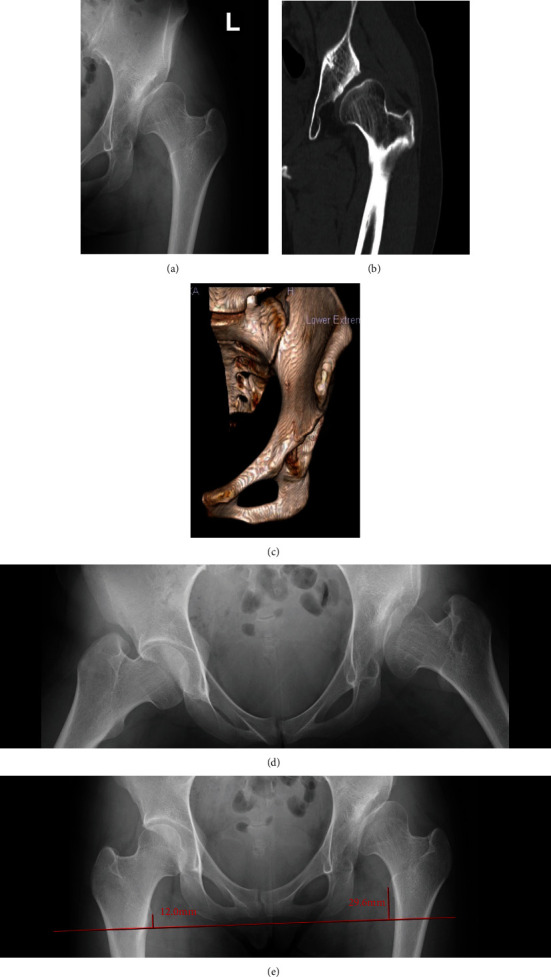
A 20-year-old woman with severe acetabular dysplasia. (a) Preoperative anteroposterior radiograph of the left hip joint, (b) preoperative CT coronal view, and (c) preoperative 3D-CT frontal view. Compatibility of the left hip joint was poor, and the femoral head of the superior acetabular coverage was insufficiently covered. (d) The abduction radiograph of the left hip shows good compatibility with the left hip joint. (e) The distance of leg length discrepancy from the center of the lesser trochanter to the bottom of the ischial tuberosity was 17.6 mm.
